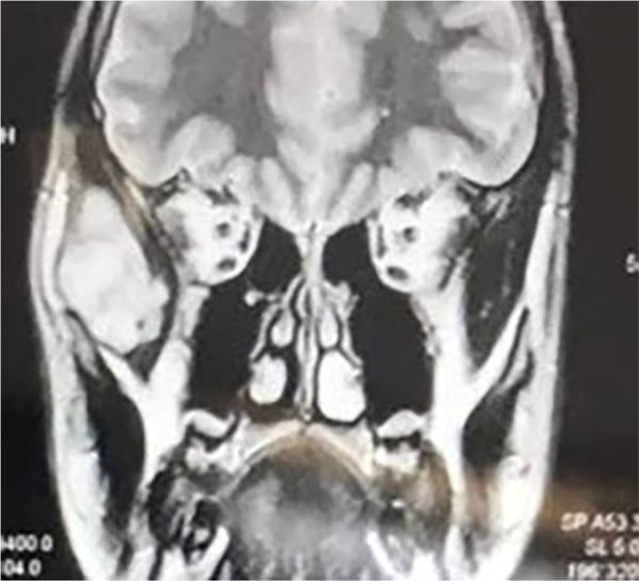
Magnetic resonance imaging (MRI) showing the lesion at the right temporal region
